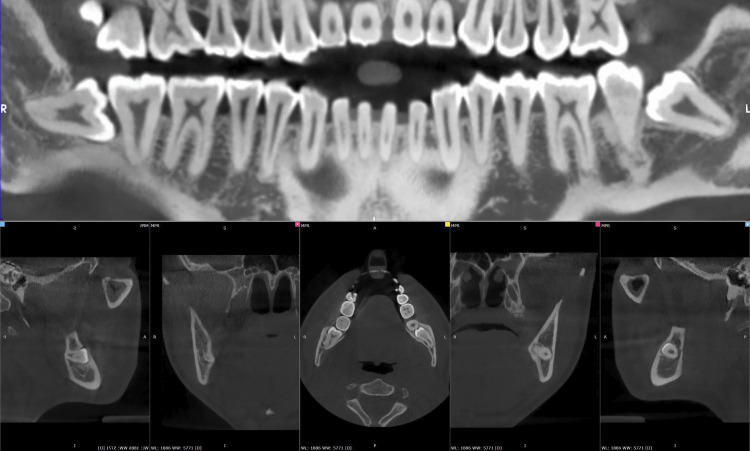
Representative preoperative CBCT assessment of MA-HM3Ms. Multiplanar reconstructions (axial, sagittal, and coronal views) depict tooth angulation, depth of impaction, root morphology, and proximity to adjacent anatomical structures, including the mandibular canal and second molar
